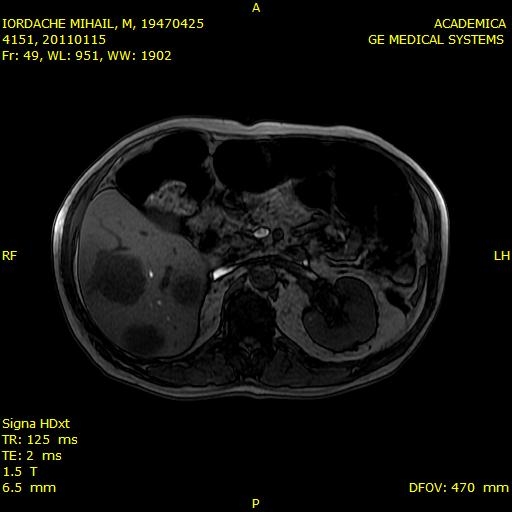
MRI revealed multiple formations in the liver parenchyma (October 2010)
